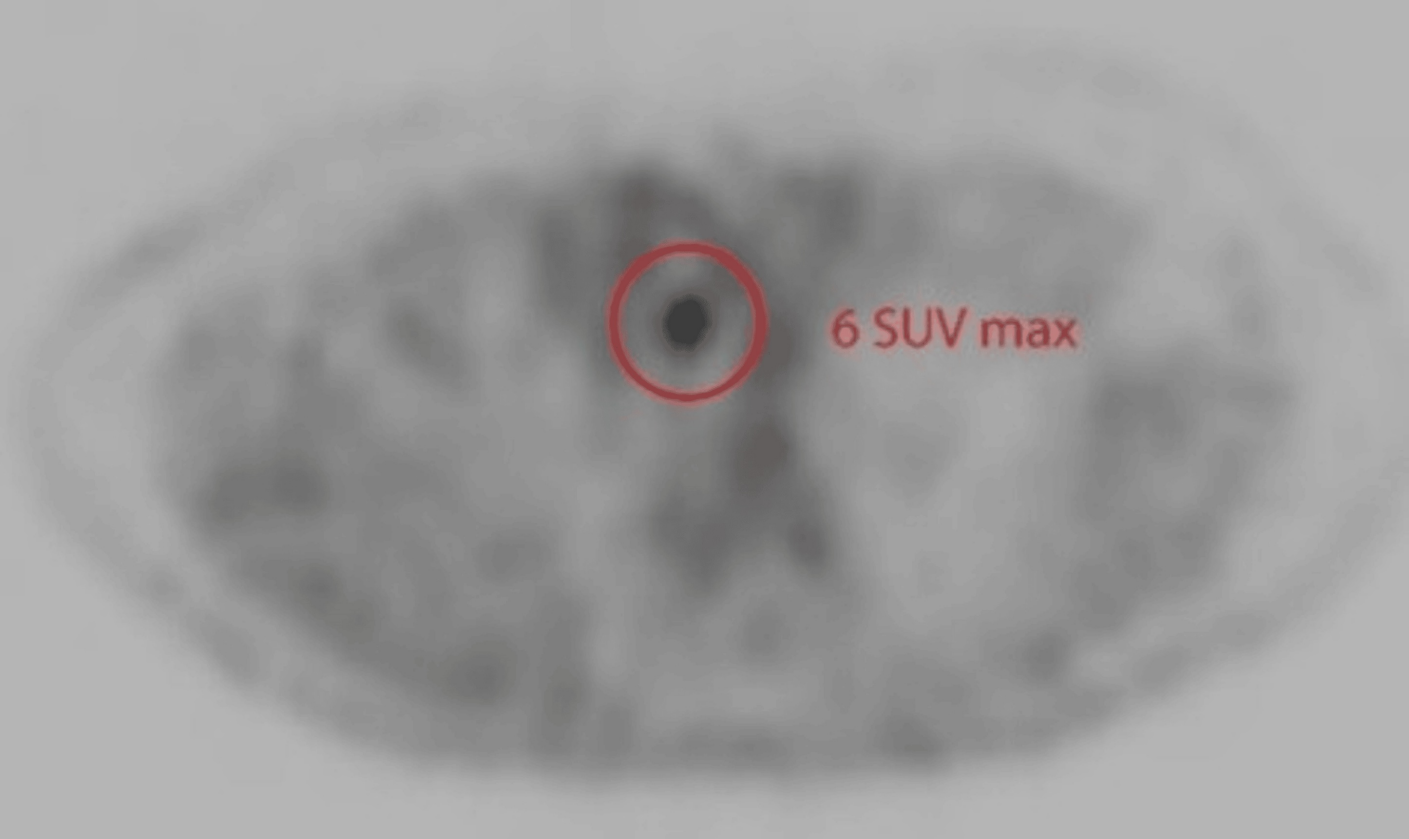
PET component showing increased metabolic activity of 6 SUV (red circle) PET: positron emission tomography; SUV: standardized uptake value
